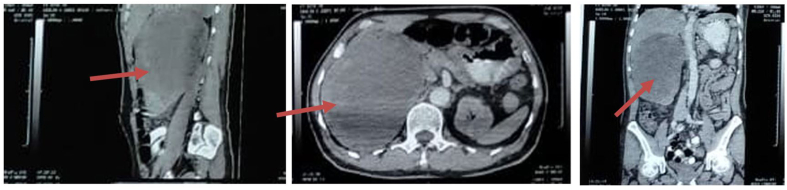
CT Scan of the Abdomen and Pelvic Showed Renal Mass (red arrow). (For interpretation of the references to colour in this figure legend, the reader is referred to the Web version of this article.)
